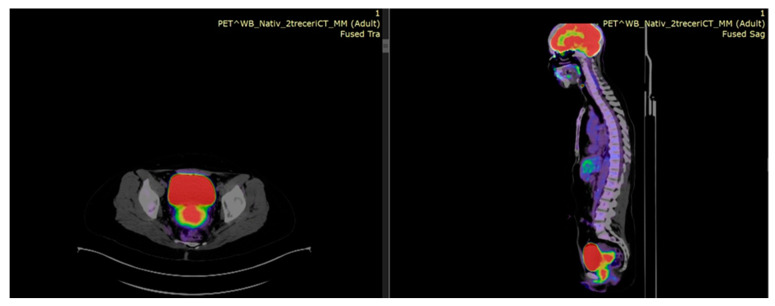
An example of pretreatment [^18^F] FDG PET–CT showing a locally advanced cervical tumor with complete vaginal invasion and bladder involvement up to urethral meatus—axial view and sagittal view.
